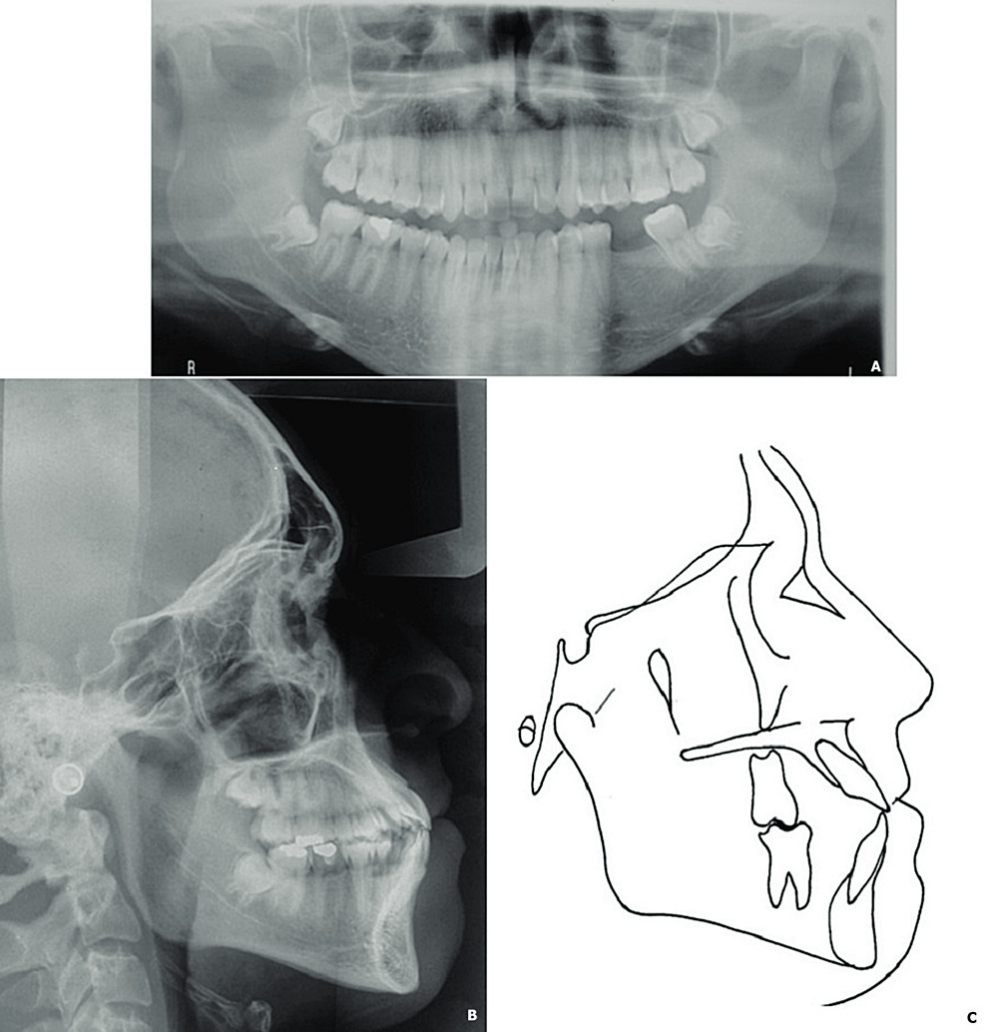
Initial radiological examination (A: panoramic radiograph; B: profile teleradiograph) and cephalometric tracing (C)
